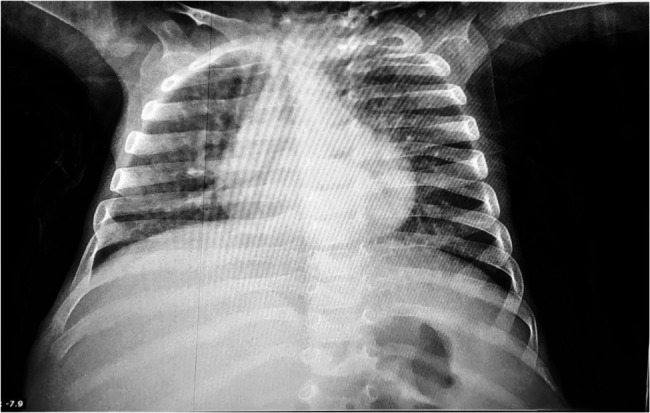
Showing the patient’s X-ray during his second pneumonia episode
